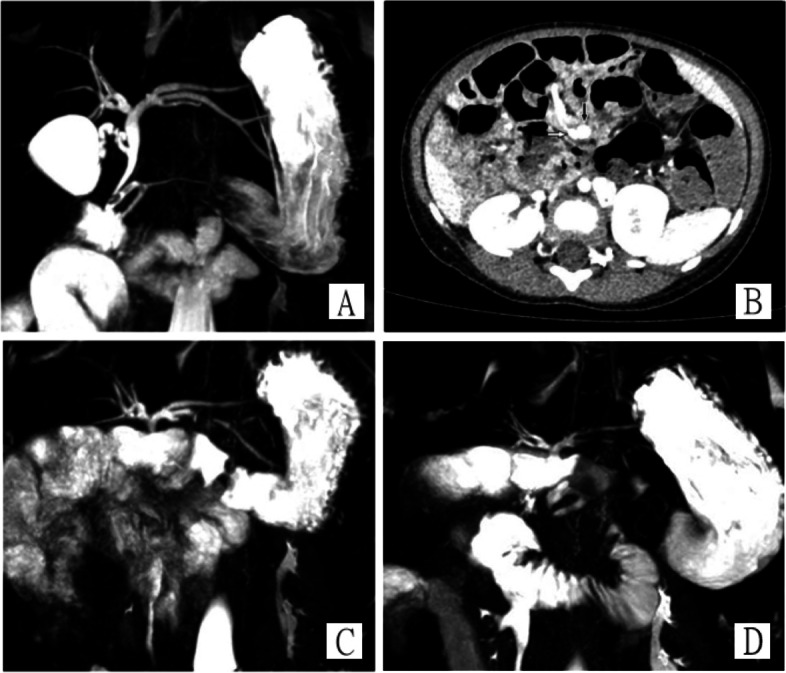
Imaging study on admission and post-operation. A MRCP showed dilated biliary system, a bile stone in the common bile duct, pancreaticobiliary maljunction, and pancreas divisum. B Abdominal CT contrast showed SMV (black arrow) positioning on the left of SMA (white arrow). C MRCP at 2 months after surgery showed the dilation of the intrahepatic bile duct is improved, the biliary-enteric anastomosis is unobstructed, no stricture, and no leak. D MRCP at 12 months after surgery
